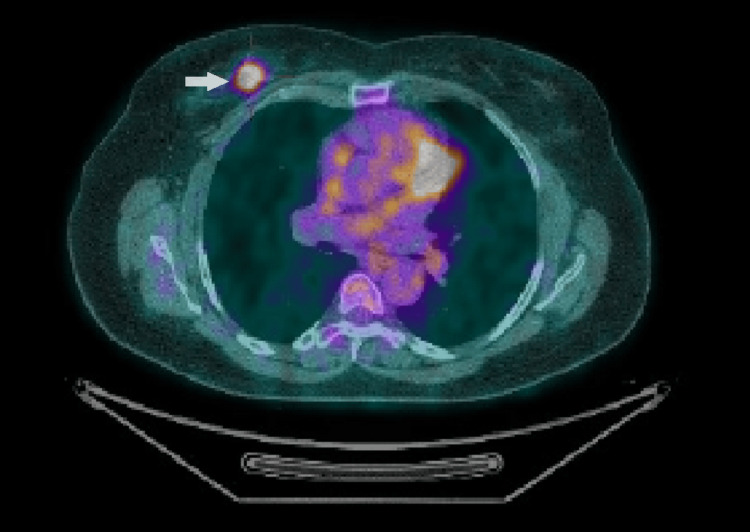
18F-FDG PET-CT before treatment revealing hypermetabolic activity in the upper inner quadrant of the breast 18F-FDG PET-CT: 18F-fluorodeoxyglucose positron emission tomography-computed tomography
